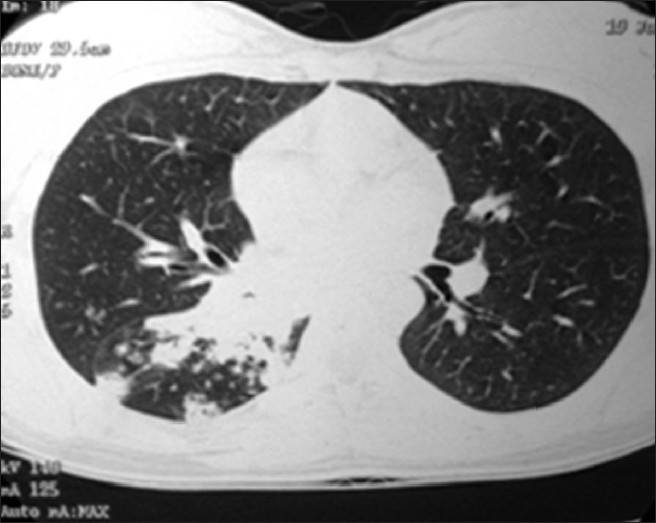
HRCT thorax showing segmental consolidation with treein-bud appearance
